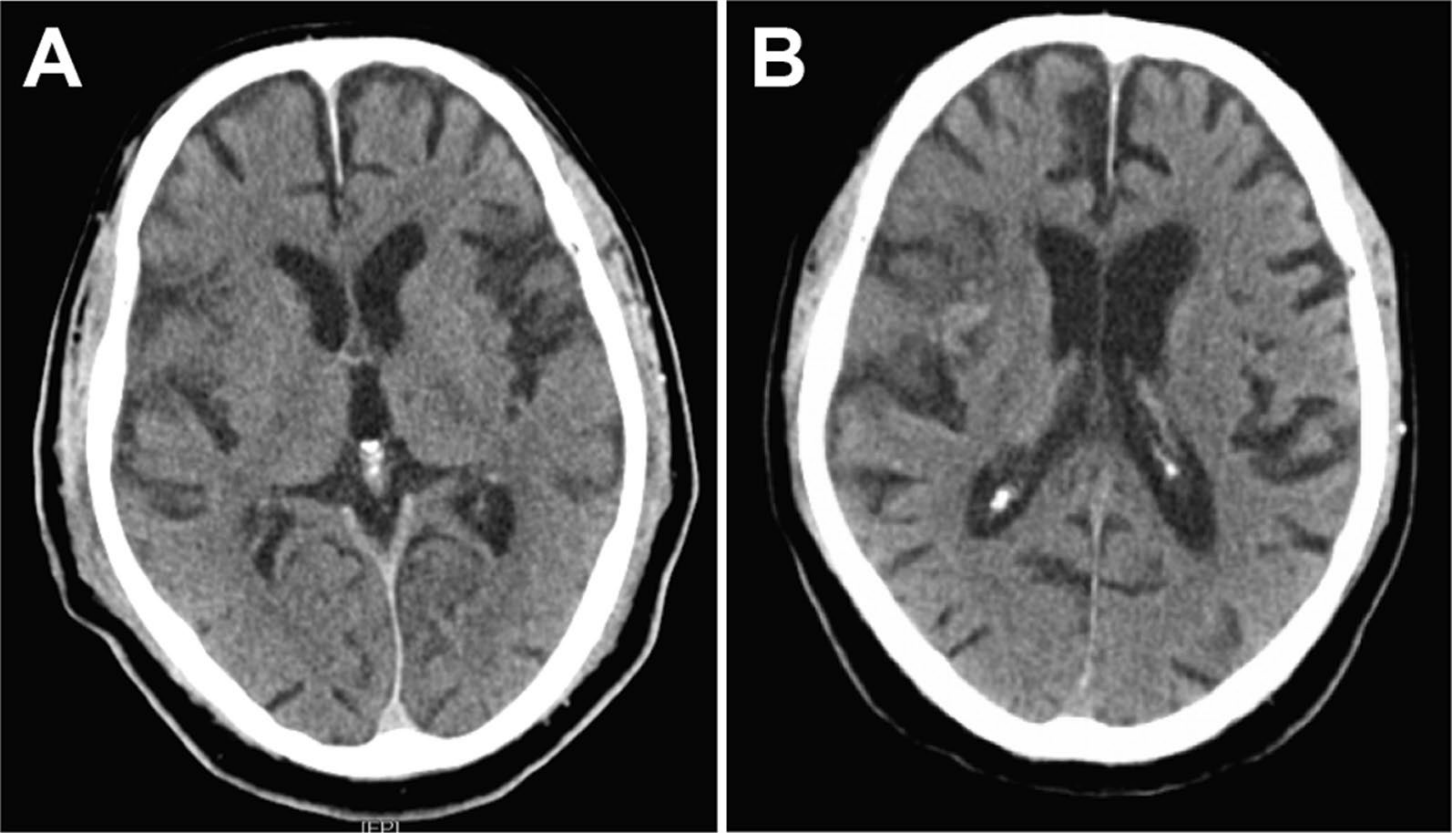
Radiological outcome in follow-up imaging. NCCT indicates non-contrast cranial CT. One day post intervention, only a small infarct demarcation was observed in the right insula (A) without any bleeding complication. Nine days post intervention, hemorrhagic transformation within the infarcted region became evident (B)
